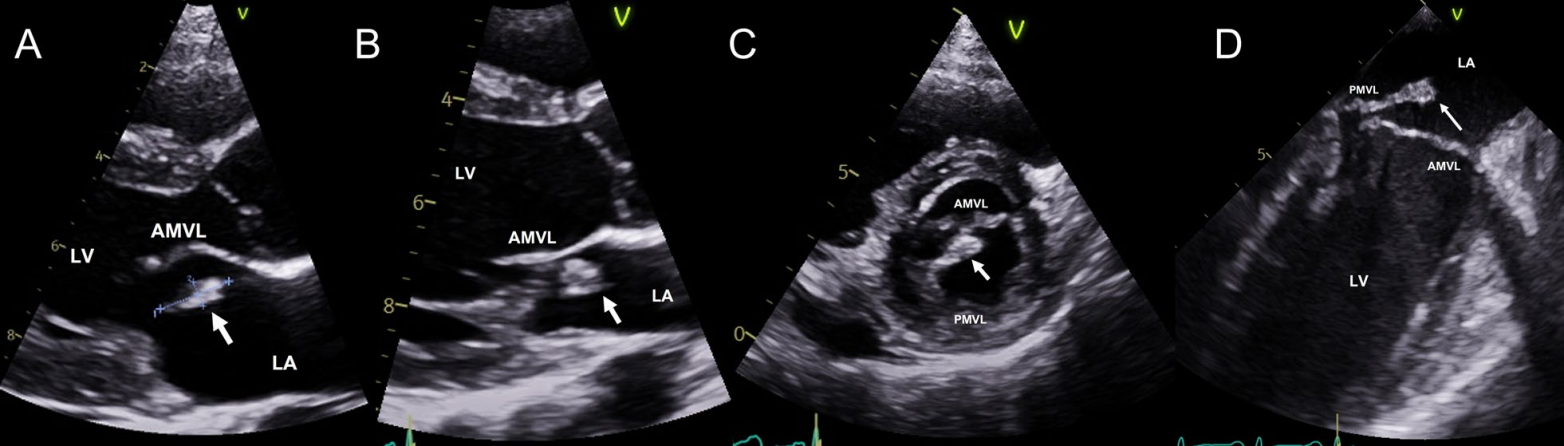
Sequential preoperative echocardiographic images. Legend: Panel A: 2D TTE parasternal long axis view showing an echogenic mass, measuring 1.48 × 0.53 cm. Panel B: 2D TTE parasternal long axis view showing vegetation on the PMVL. Panel C: 2D TTE parasternal short axis view showing vegetation on the medial (P3) segment of the PMVL. Panel D: 2D TOE ME two-chamber view showing vegetation attached to the PMVL. 2D, two-dimensional; TTE, transthoracic echocardiography; PMVL, posterior mitral valve leaflet; TOE, transesophageal echocardiography; ME, mid-esophageal
